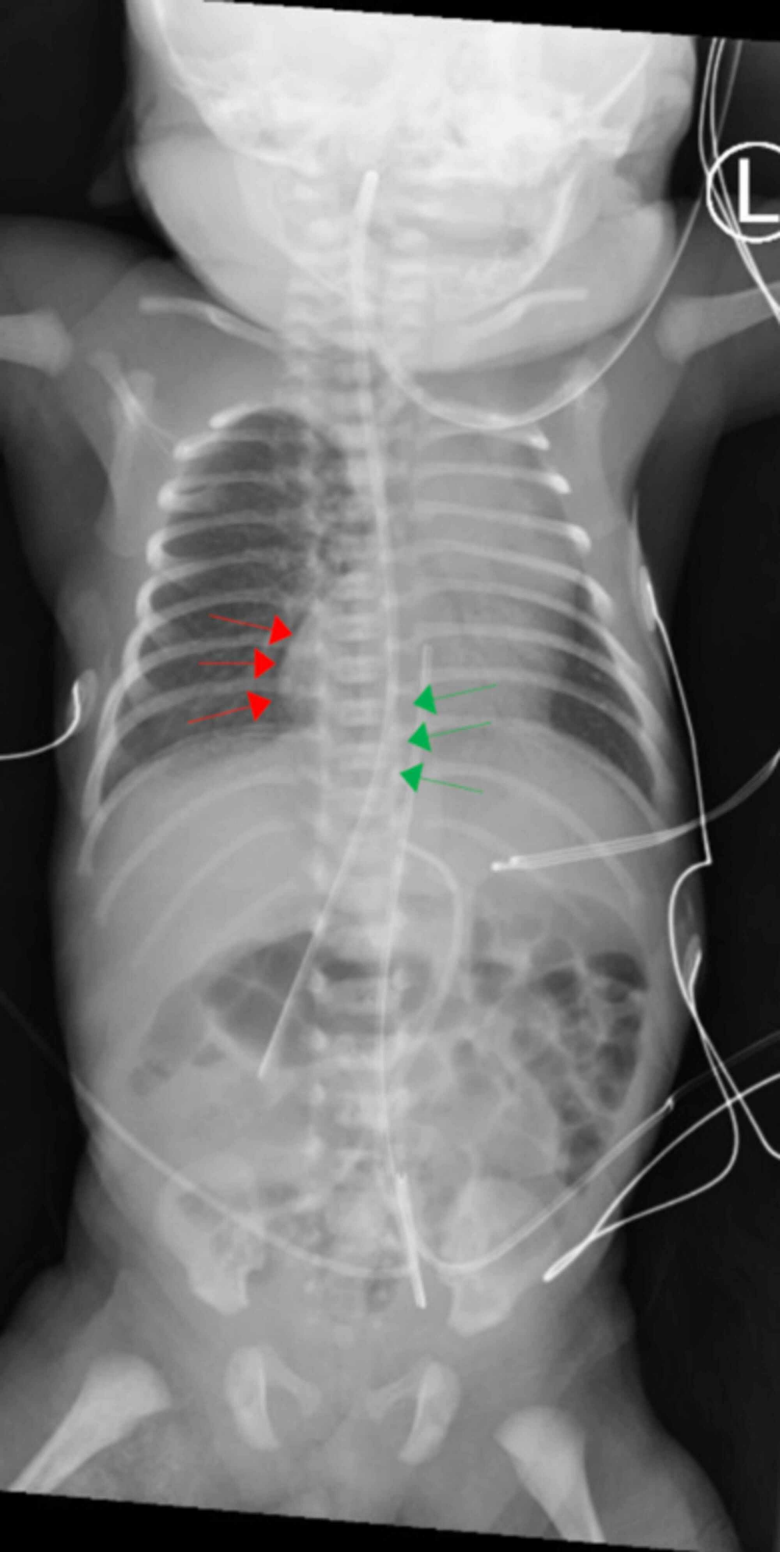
Mobile chest and abdominal X-ray indicate entrance of the gastric tube (green) to the right side of the patient’s body. The red line indicates the apex of the heart on the patient's right side. A gastric air bubble is apparent on the patient’s anatomical right side. Also the liver appears midline. There is no evidence of gut malrotation.
